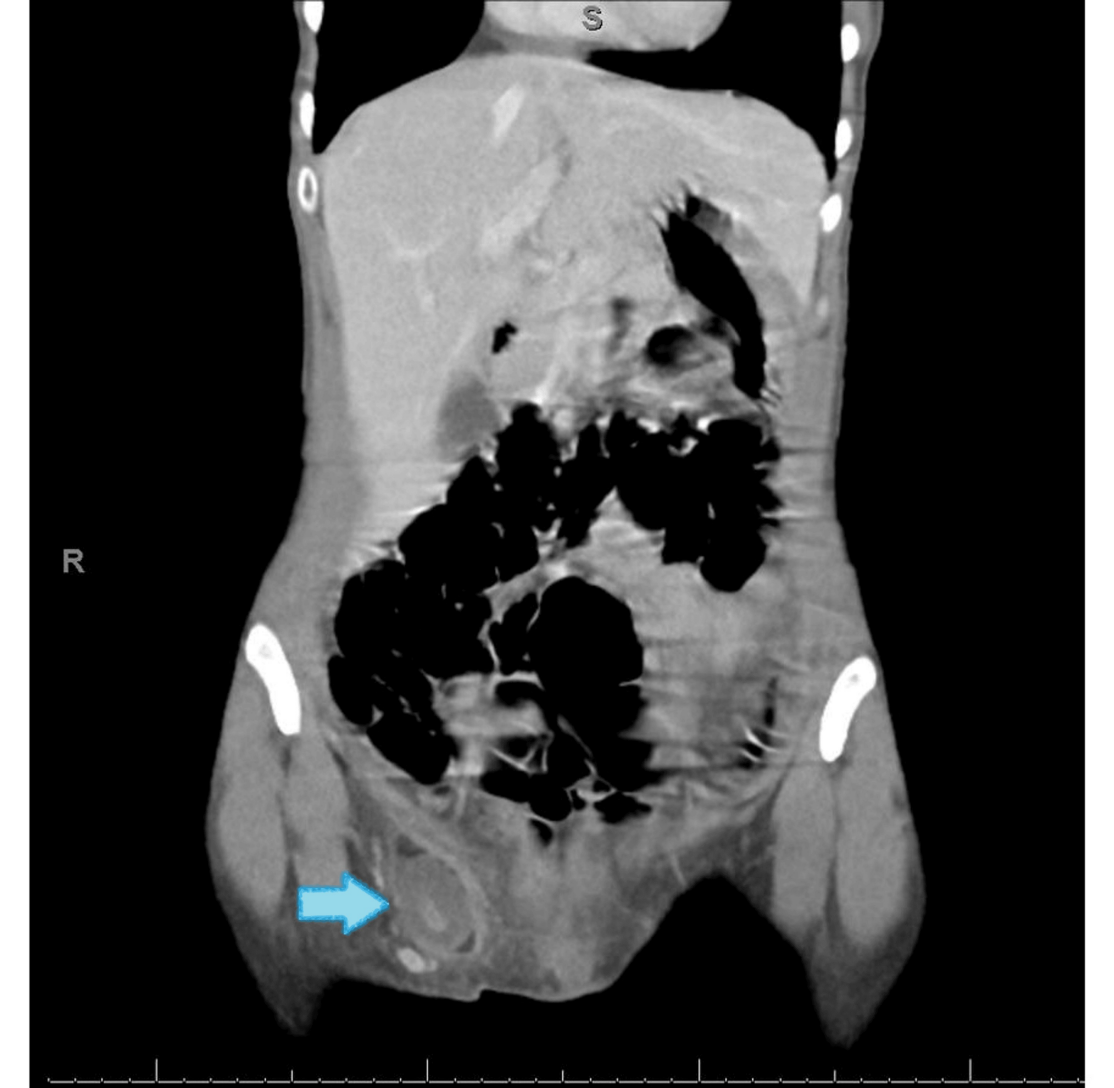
Abdominal CT scan with contrast revealing a femoral hernia on the right side containing the inflamed, dilated, 7 mm appendix, swirling fluid, and surrounding stranding indicative of inflammatory changes.
